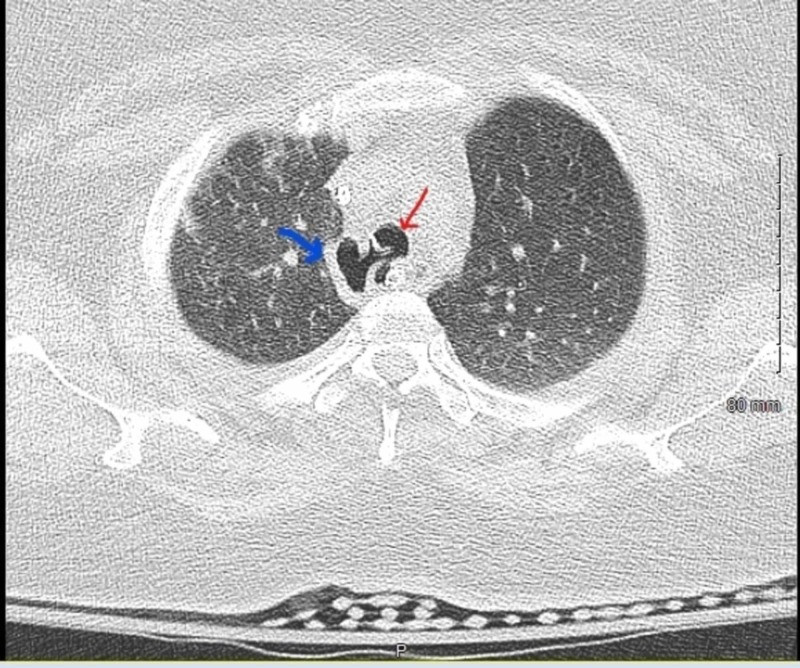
Computed tomography of the chest showing tracheal diverticulum at the right posterior lateral tracheal wall indicated by the blue arrow and trachea indicated by the red arrow
